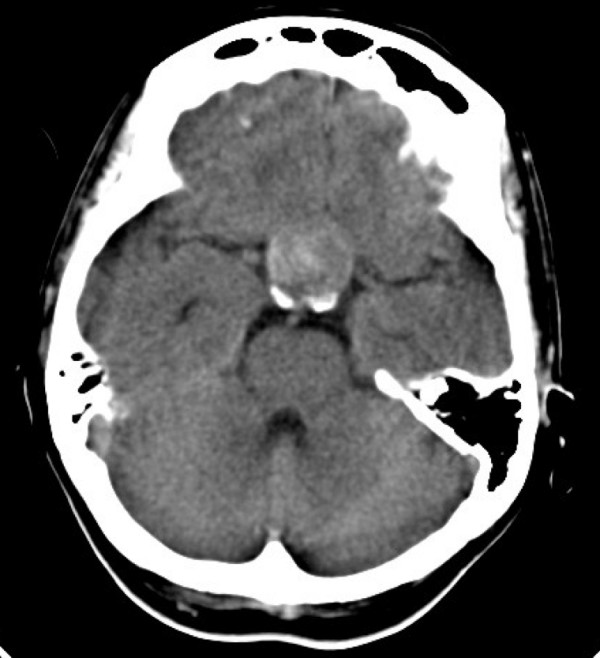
CT scan of the pituitary demonstrating haemorrhage. Patchy enhancement of the pituitary mass is seen indicating haemorrhage within the pituitary macroadenoma.
